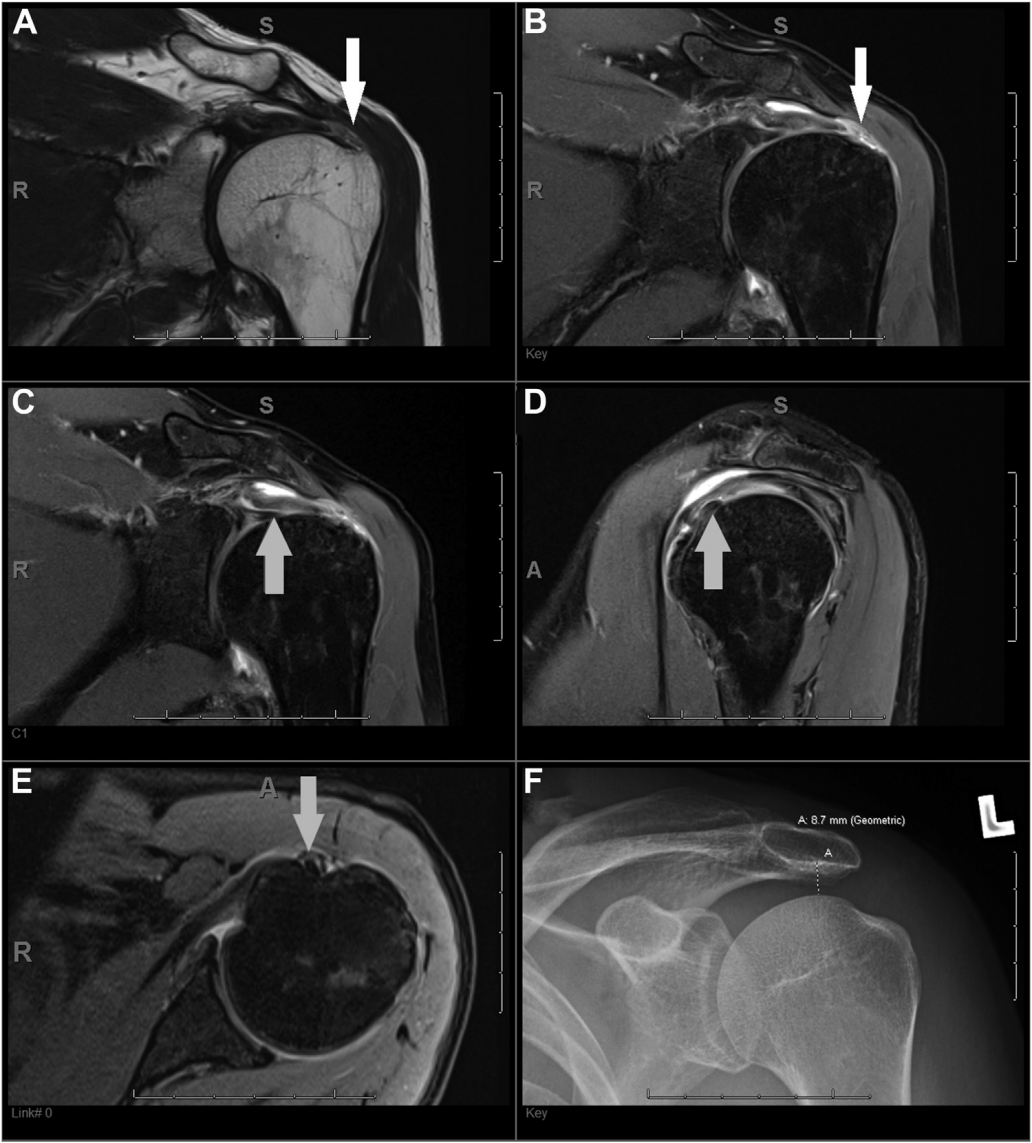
A 3T MRI scan of the left shoulder joint on a 35-year-old male patient using routine protocol. (A) Coronal T2-weighted image and (B) coronal proton density fat saturated image demonstrating a full thickness tear of the supraspinatus tendon insertion (*white arrows*). (C) Coronal proton density fat saturated image, (D) sagittal proton density fat saturated image, and (E) axial proton density fat saturated image demonstrating an intact biceps anchor and pulley within the bicipital groove (*gray arrows*). (F) A frontal radiograph of the left shoulder showing a maintained acromiohumeral distance (8.7 mm). *MRI*, magnetic resonance imaging.
